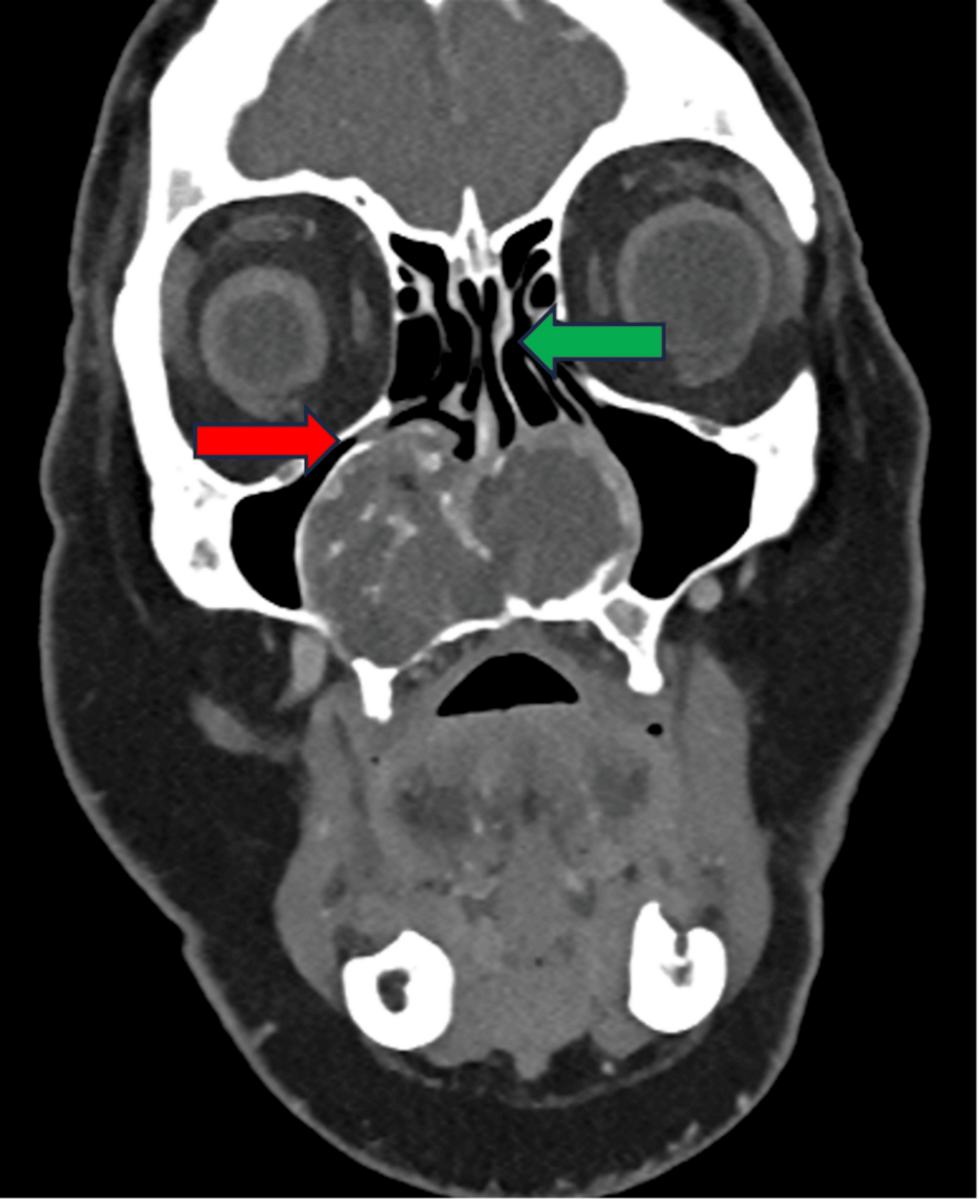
Coronal contrast-enhanced CT of sinonasal chondrosarcoma The image shows a lobulated mass centered on the anterior-inferior nasal septum, expanding into both maxillary sinuses. The lesion spares the orbital floor (red arrow) and the ethmoidal labyrinth (green arrow), confirming the absence of orbital or ethmoidal invasion CT: computed tomography
